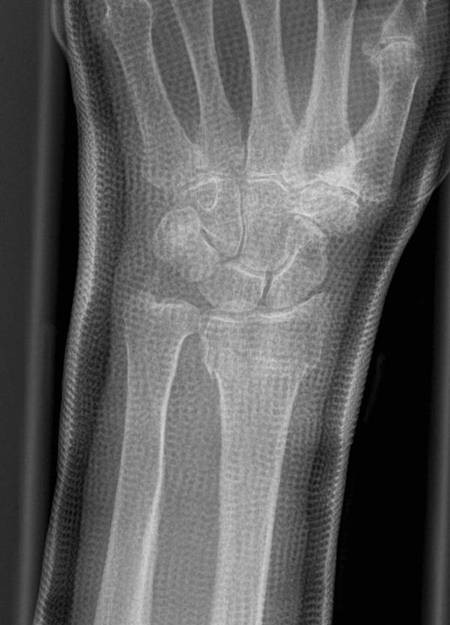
Post-reduction radiograph of the wrist. Mild shortening of radial length in the anteroposterior view after casting
